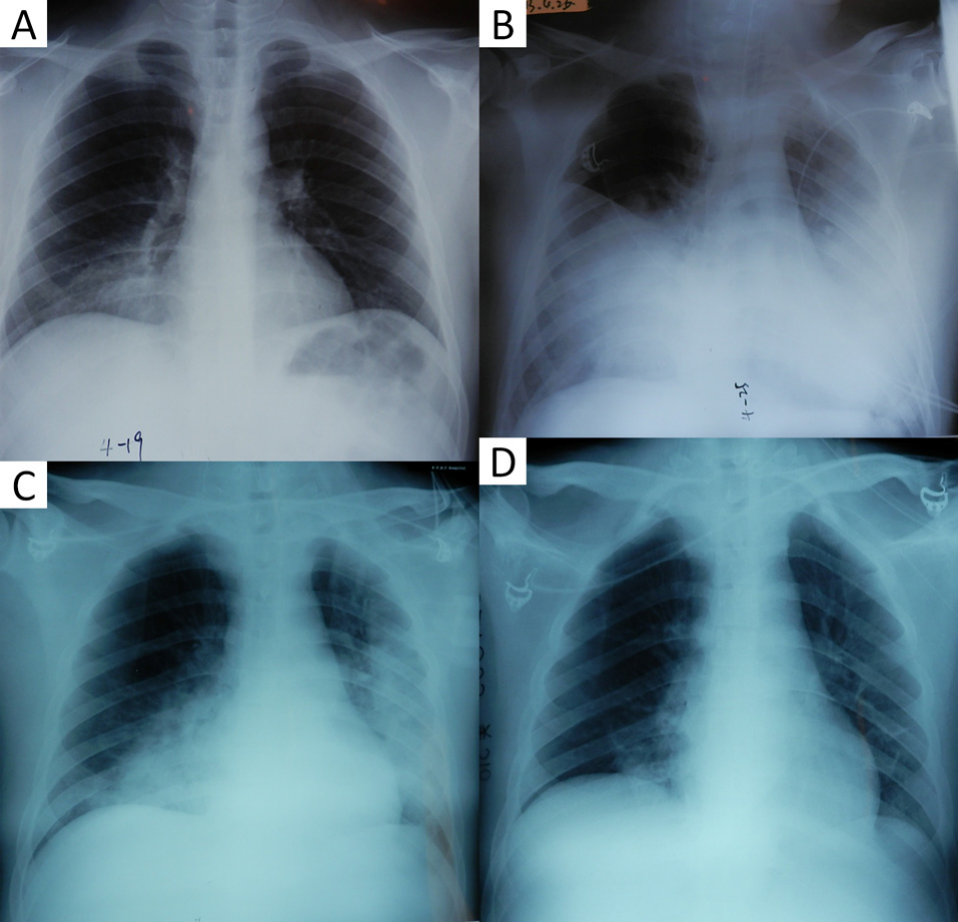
23 year-old man with adenovirus type 55 pneumonia. (A), Initial chest radiograph (day 2 after the onset of illness) shows focal consolidation in the right lower lobe. (B), Chest radiograph (day 8 after the onset of illness) reveals rapid progression, widespread consolidations in both lungs. (C), Consolidations in both lungs have begun to decrease by day 15 after onset. (D), Consolidations in both lungs have further decreased by day 18 after onset.
